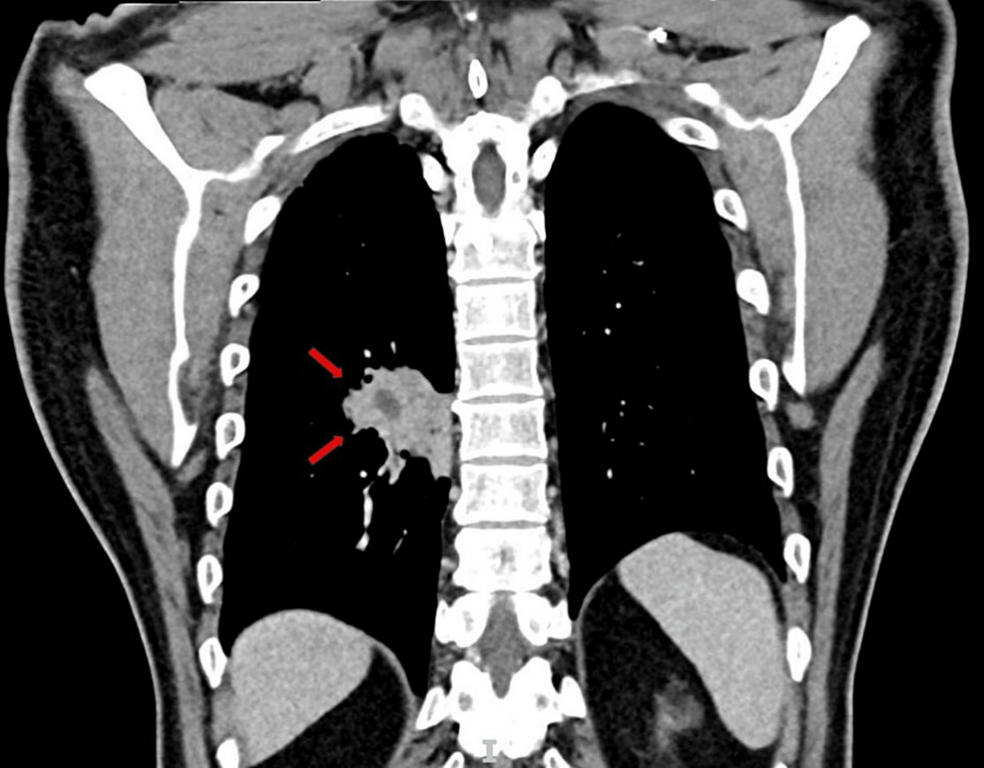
Computed tomography of the thorax coronal view with new mass-like consolidation in the right lower lobe.
